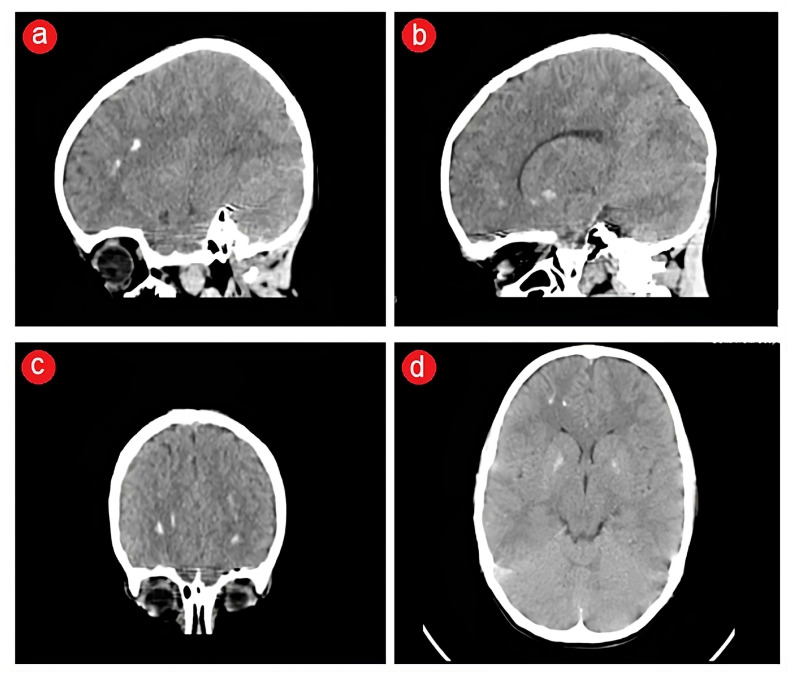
CT scan displaying calcifications in the frontal cortex and basal ganglia, with no indications of infarction, hemorrhage, or mass effect, as observed in sagittal (a,b), coronal (c), and axial (d) views.
